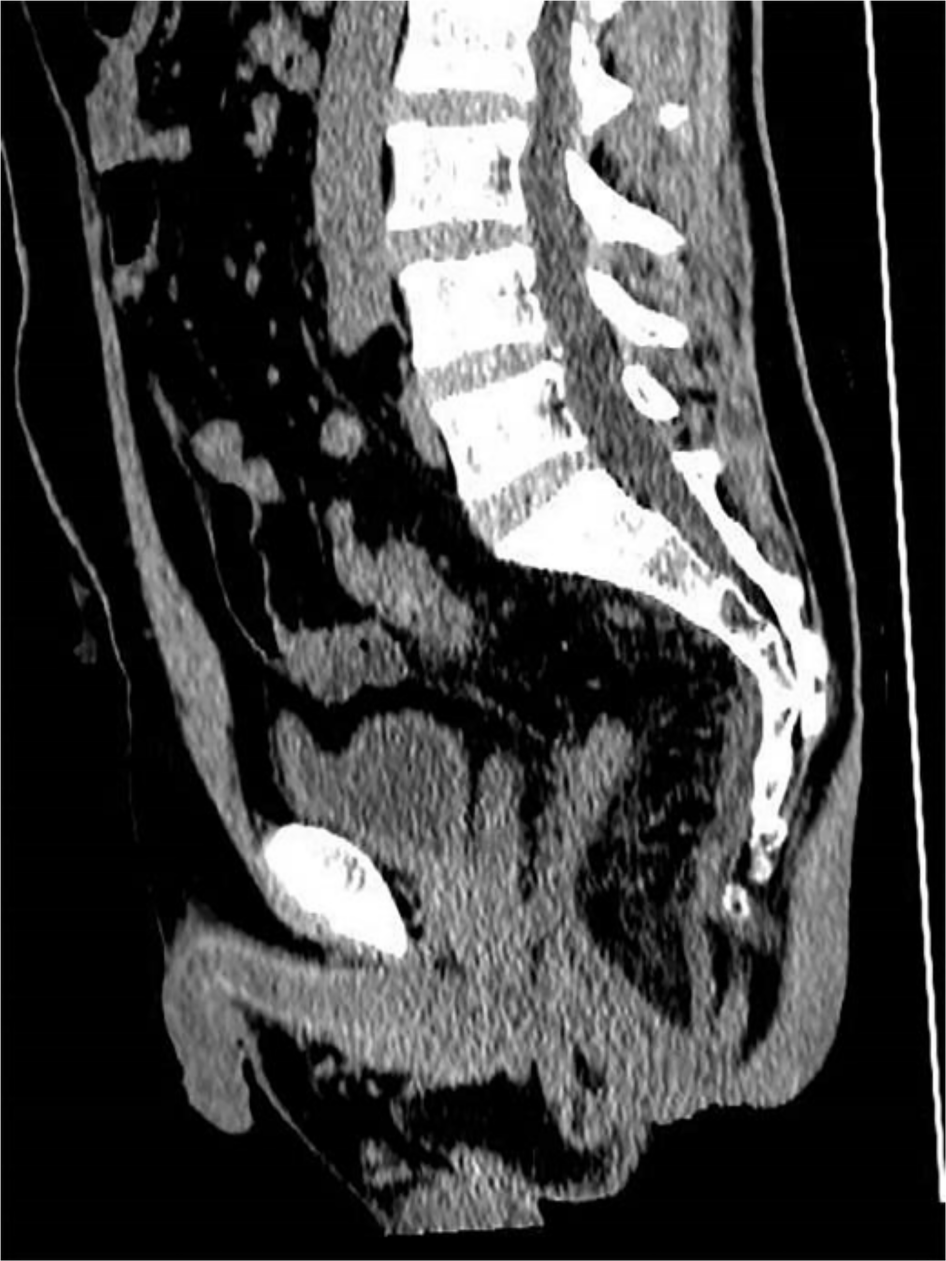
CT image after removing the trans-anastomotic drainage tube (postoperatively day 7).
